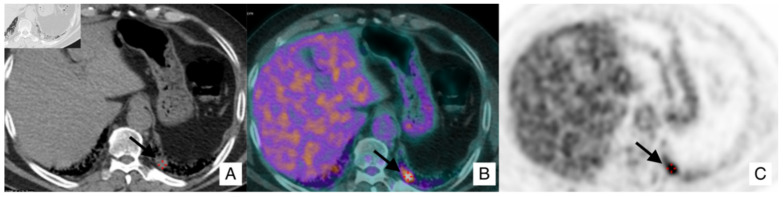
PET-CT (A–C) of the same patient of Figure 6 and Figure 7. The exam demonstrates an increased activity (uptake of 6.84) in the nodular lesion, which is clearly depicted (black arrow) in the axial CT image (A), in the fusion colour image (B) and in the black and white PET map (C).
